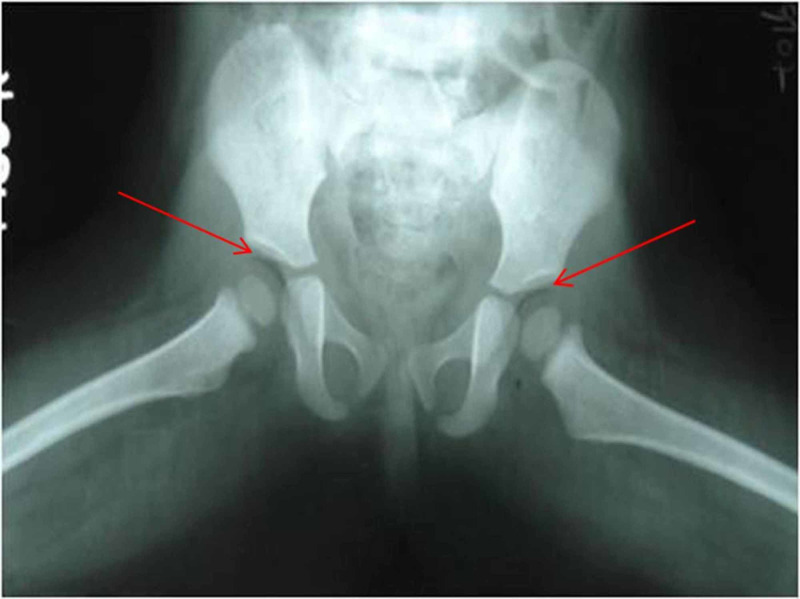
Frog-leg position radiograph of hip joints in an eight-month infant showing pneumoarthrosis
